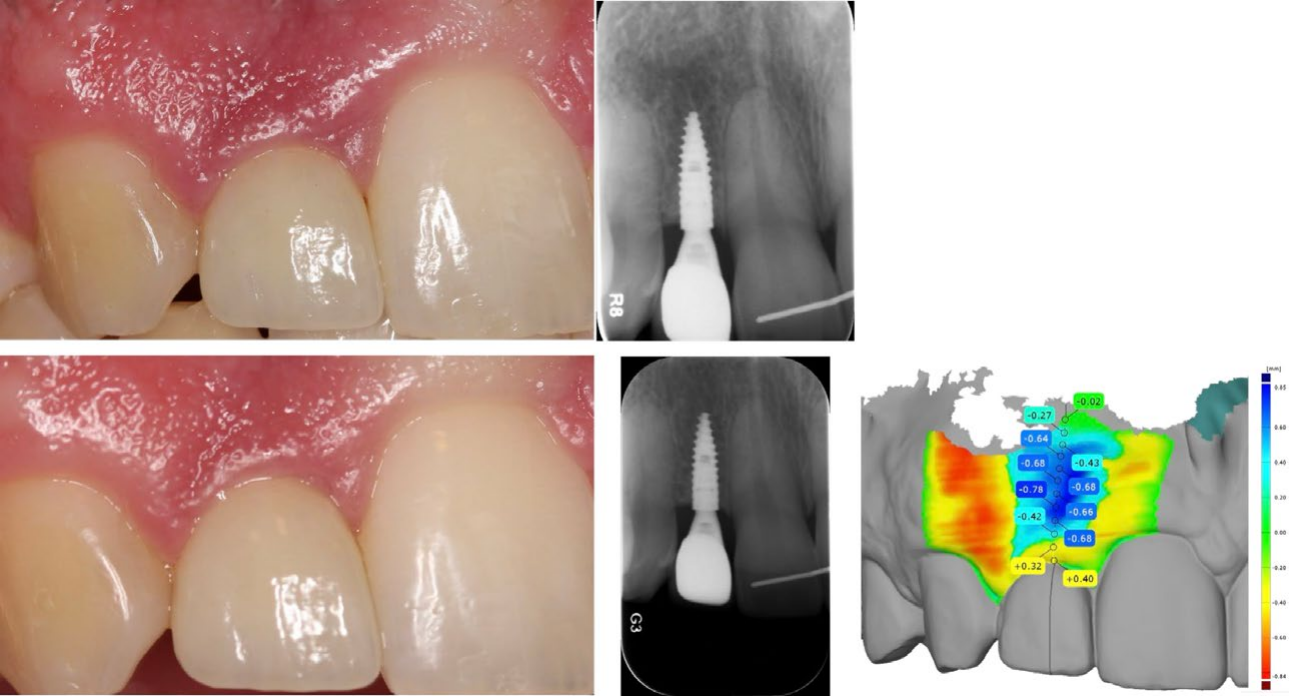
Clinical and radiographic presentation of a Ø2.9 mm implant at the 1‐ (a) and 5‐year (b) follow‐up along with the identified profilometric concave profile (c).
